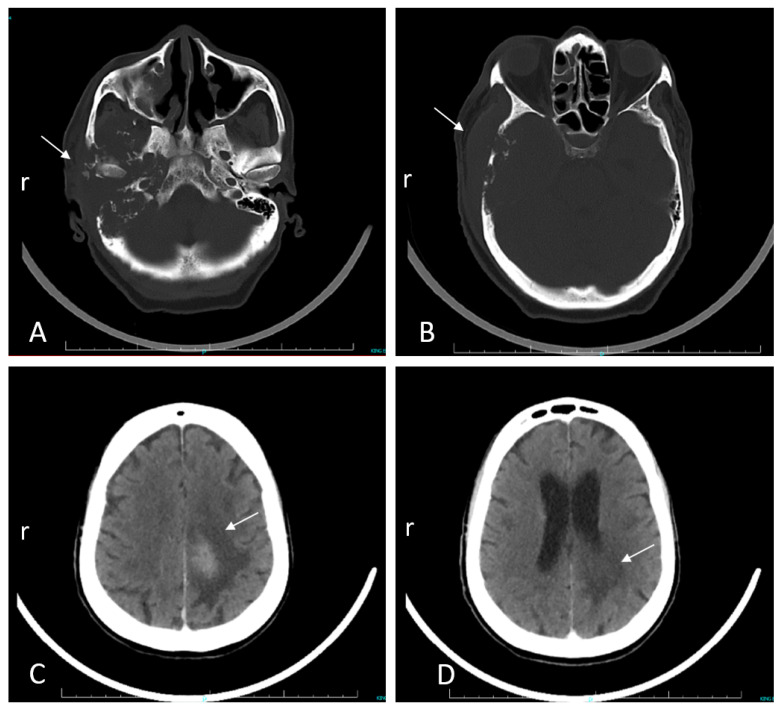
Antemortem computed tomography (CT) brain scans. Arrows indicate respective lesions. (A) Non-enhanced CT showing bony destruction and erosion of the right-side mastoid bone and facial nerve canal, the petrous and squamous temporal bones, and zygomatic process. (B) Non-enhanced CT at orbital cavity level showing extracranial soft tissue swelling and temporal bone erosion. (C) Contrast-enhanced CT demonstrating the hyperdense left parietal parenchymal brain lesion with surrounding edema that extends inferiorly, shown in (D).
